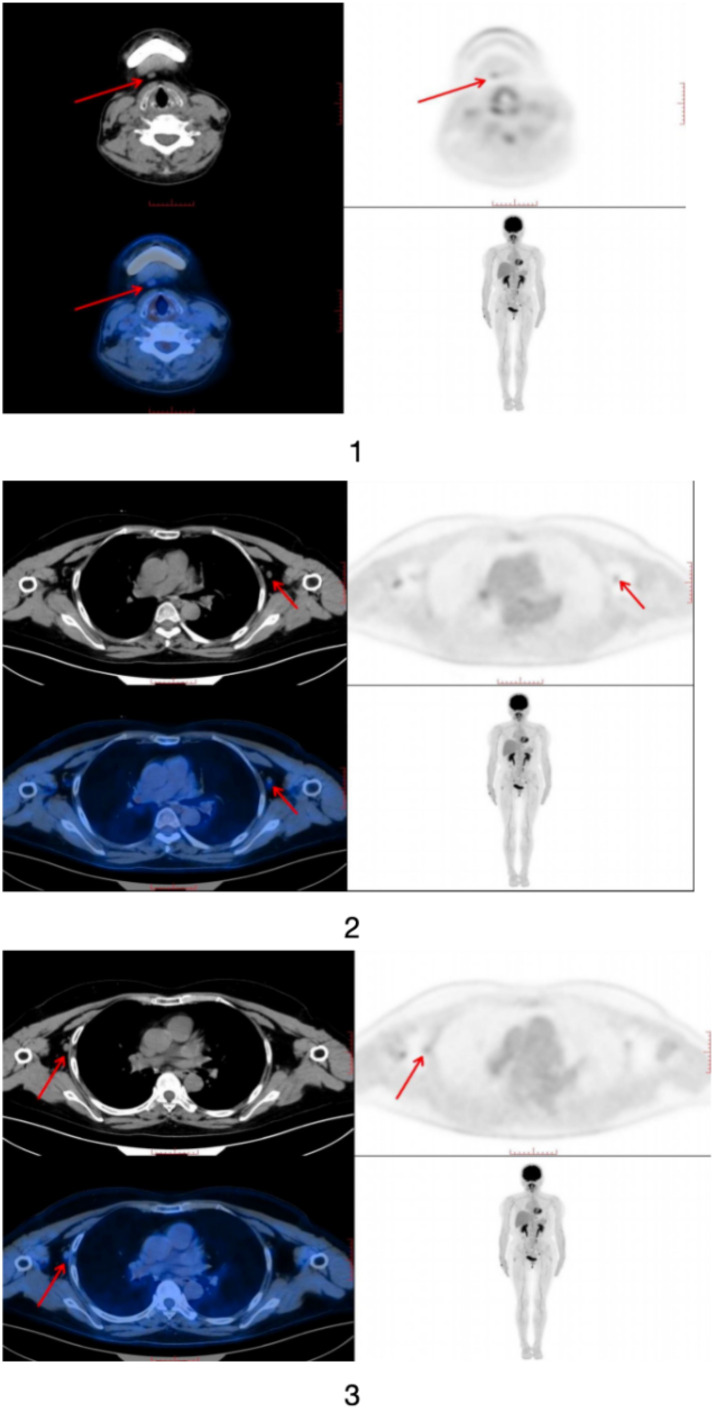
PET/CT images of the patient.
